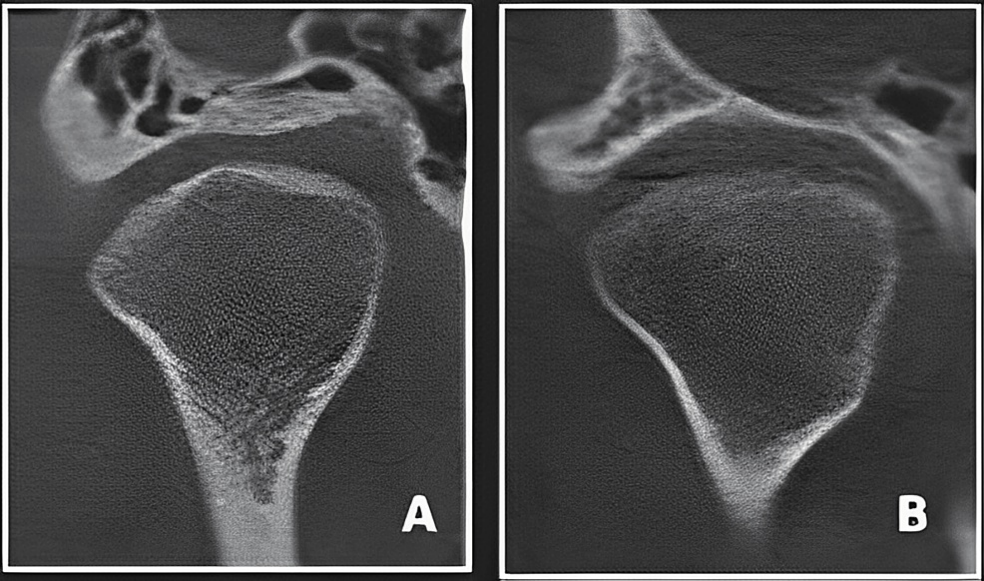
TMJ CBCT image: (A) Lateral aspect. (B) Axial aspect. CBCT, cone beam computed tomography; TMJ, temporomandibular joint
